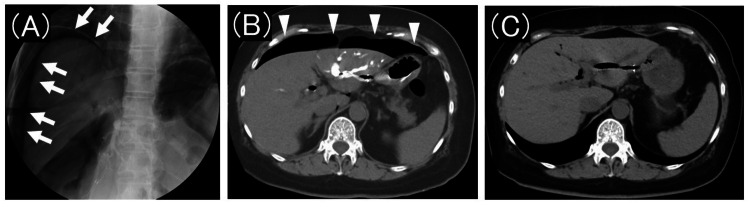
Fluoroscopic and computed tomography images of pneumoperitoneum (A) During endoscopic retrograde cholangiopancreatography, a large amount of gas (arrow) appeared between the liver and diaphragm on fluoroscopic images. (B) A computed tomography scan immediately after endoscopic retrograde cholangiopancreatography reveals a substantial amount of free air (arrowhead) within the peritoneal cavity. No intraperitoneal leakage of the contrast medium is observed. (C) A computed tomography scan conducted one week after the procedure demonstrates complete resolution of the free air within the peritoneal cavity.
